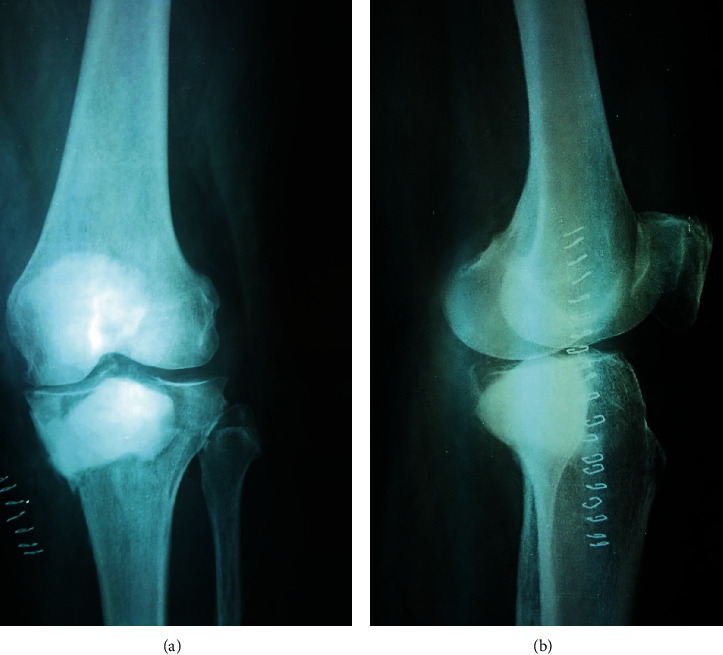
Immediate postoperative radiograph ((a) anteroposterior, (b) lateral) showing the complete containment of the PMMA bone cement within the lytic cavity.
